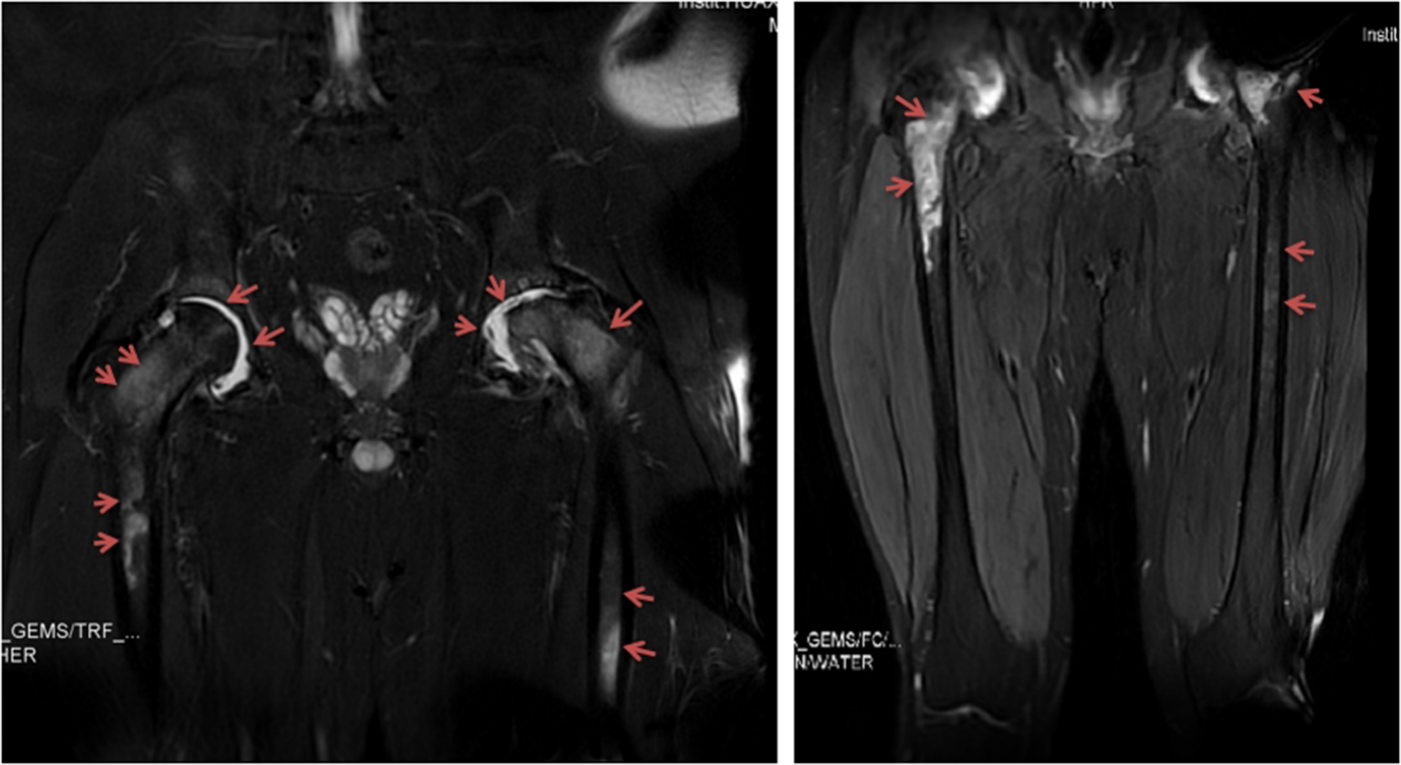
Patient 5: magnetic resonance imaging of the hip joint indicated joint effusion and periarticular bone and femur marrow signal changes. Images were taken six months after the onset of hip pain and fever.
